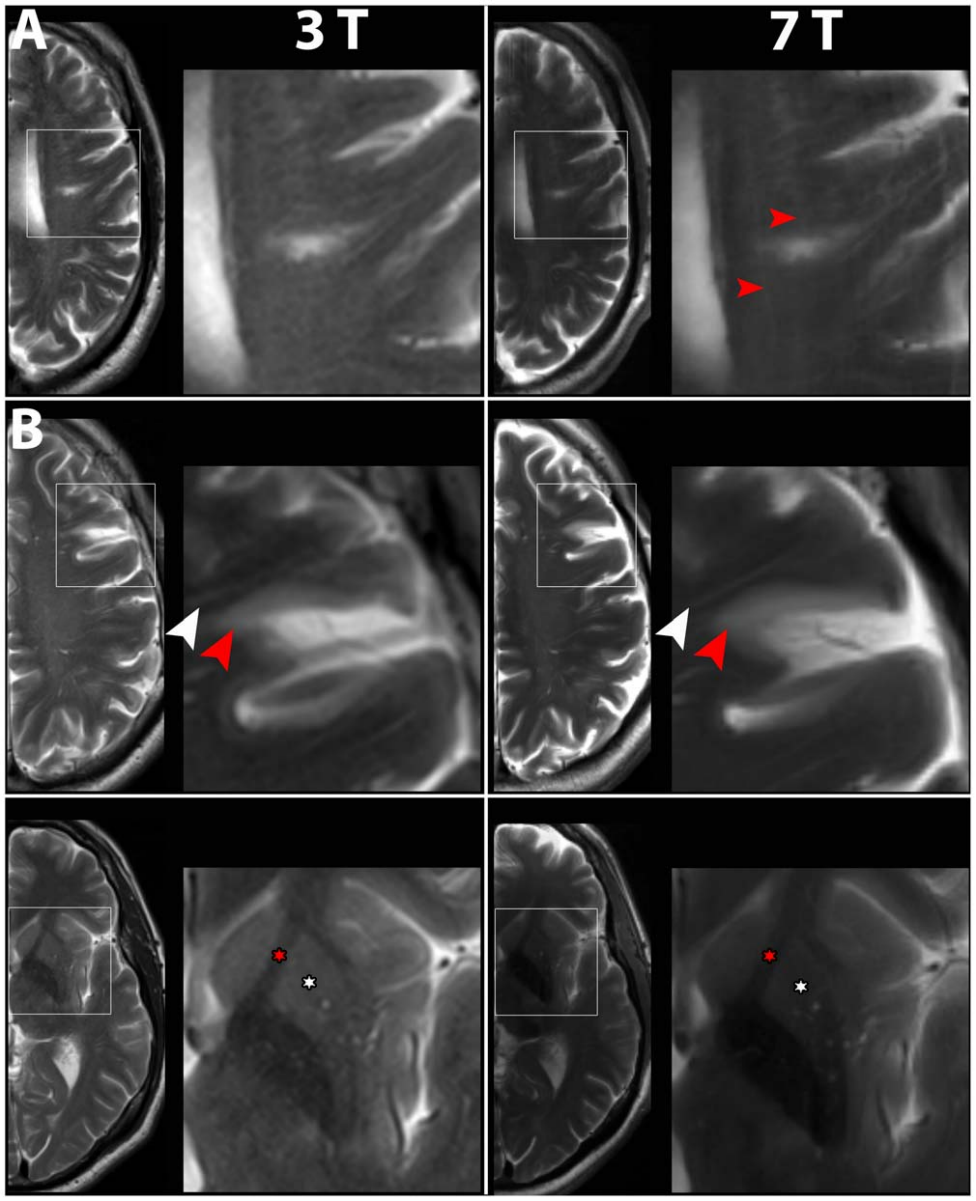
Comparison of T2-weighted imaging performed at 3 T and 7 T. Boxed areas are shown at higher magnification. A) In patient No. 3, artifacts were present (red arrowheads). Contrast and detail level of the lesion (white arrowhead) and of Virchow-Robin spaces were not higher at 7 T. B) In contrast, in patient No. 1 no artifacts were present. Virchow Robin spaces (white arrowheads) were depicted in higher detail at 7 T and the delineation of the lesion (red arrowheads) from healthy tissue was higher at 7 T. C) Same patient as in B). Also in the region of the deep nuclei T2-weighted imaging at 7 T showed better delineation, e.g. between deep nuclei (red asterisks) and fibre bundles of the internal capsule (white asterisks).
